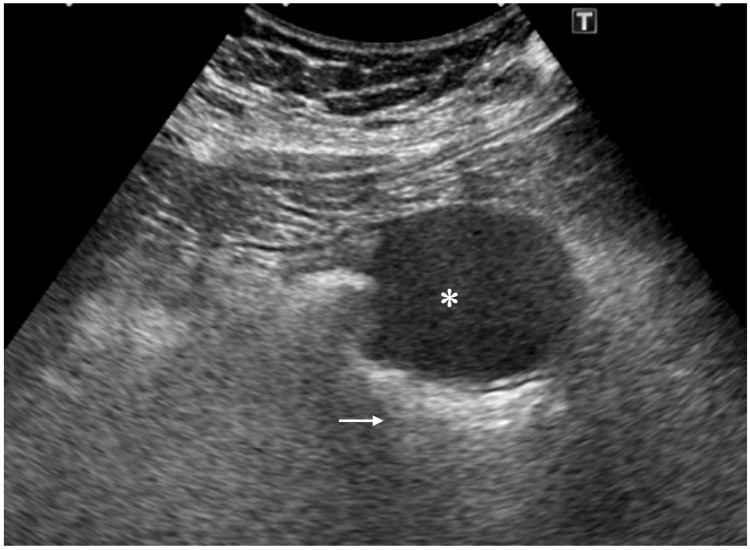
Ultrasound findings showing a mass (asterisk) with clear borders, homogenous and very low internal echoes, and enhanced posterior echoes (arrow).
